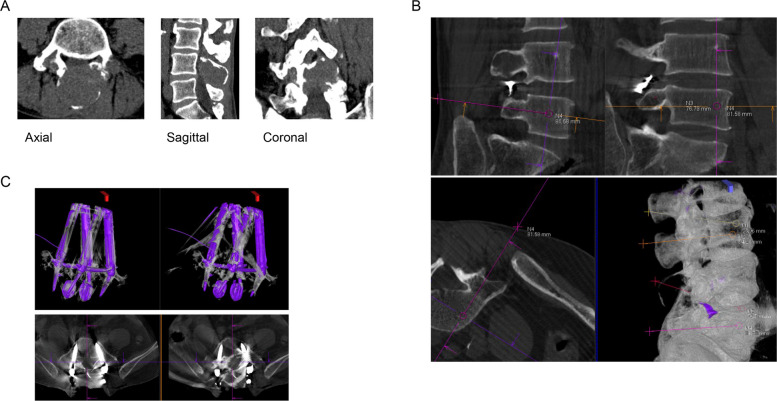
Presentation of case 3. A Pre-operation computed tomography (CT) images. B Planning determination of needle guidance on workstation. C. Post-operation CT and three-dimensional images
